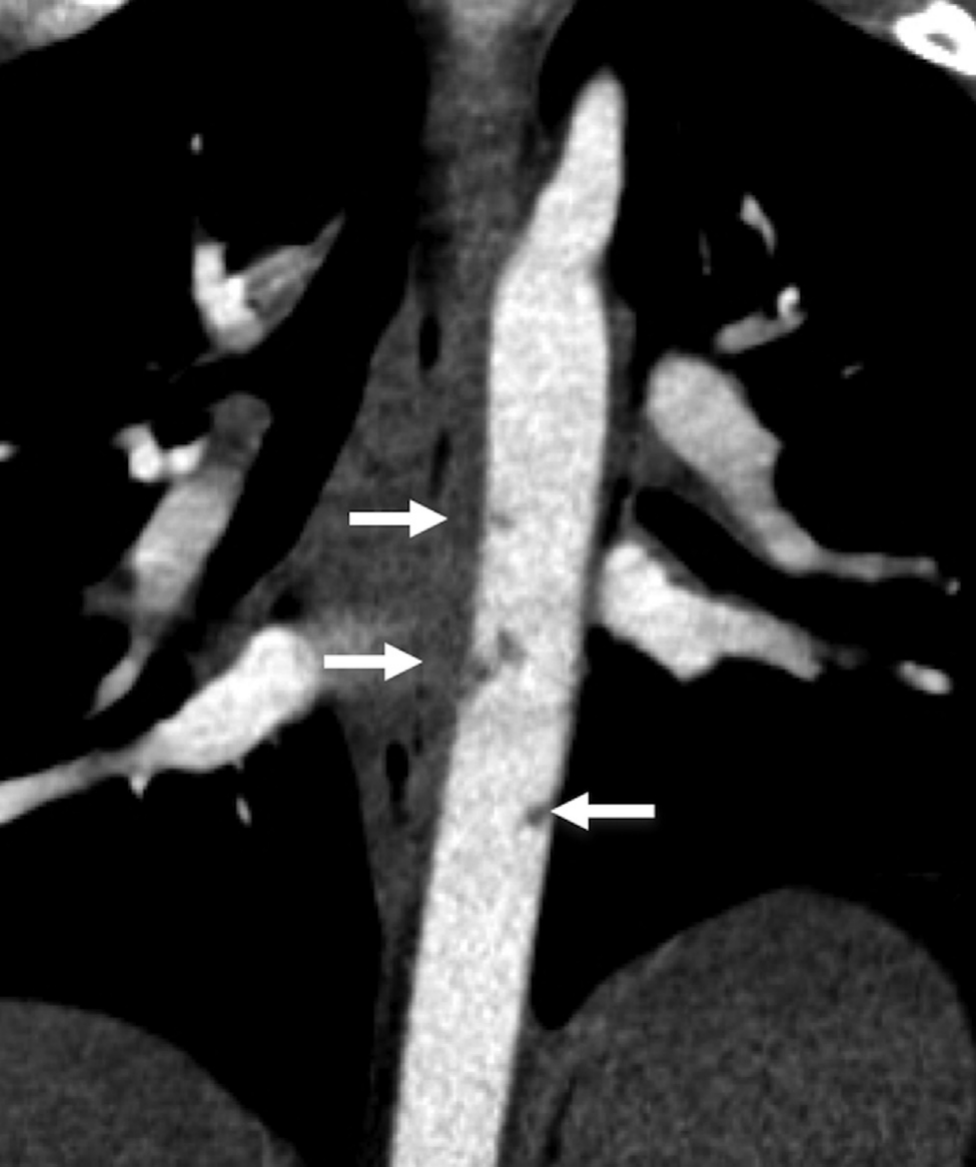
Coronal multiplanar reconstruction computed tomography angiography demonstrates multifocal minimal aortic injury in a trauma patient (white arrows). All injuries resolved on follow-up CTA imaging in 30 days.
